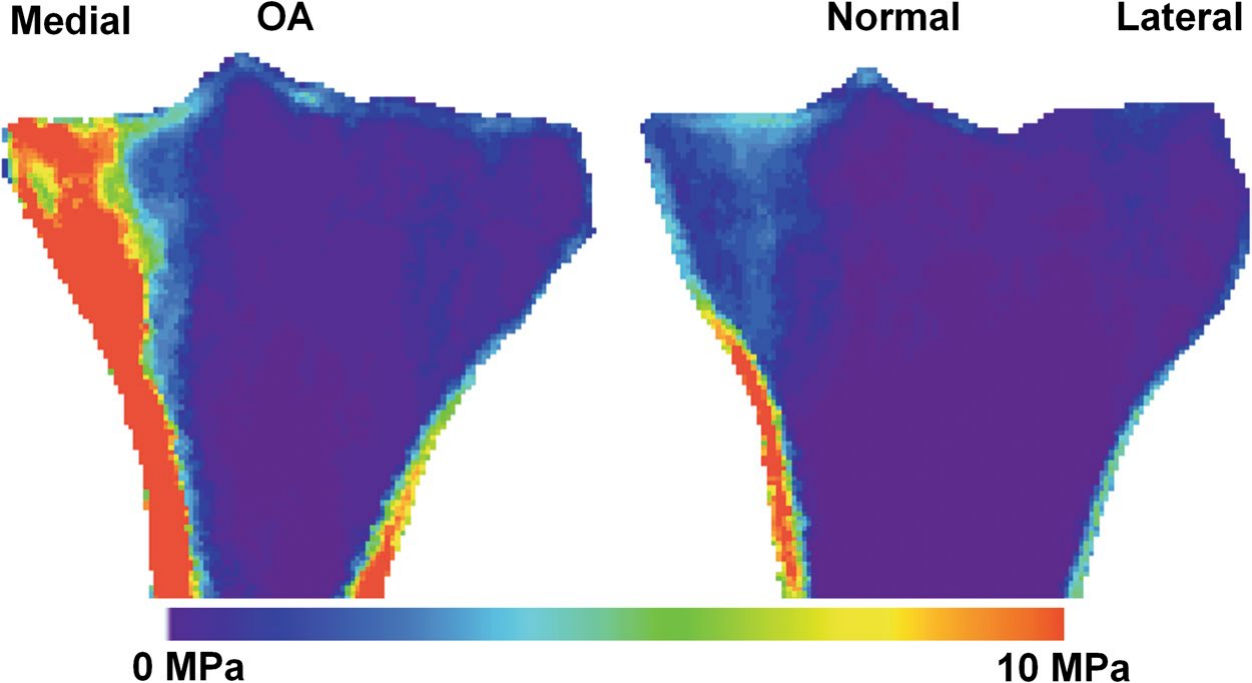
Min principal stress comparison between OA and normal proximal tibia. Min principal stress of OA and normal proximal tibia are demonstrated in coronal section of CT image. Red indicates high stress while blue is low stress.
